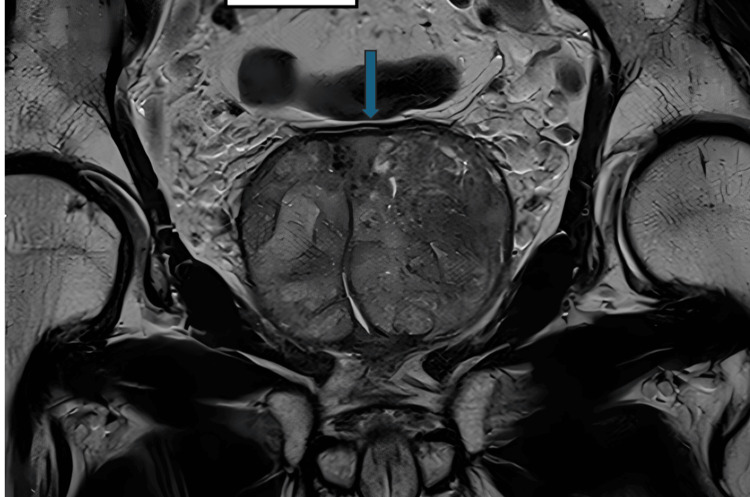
Coronal view of the MRI demonstrating bi-lobar enlarged prostate (blue arrow)
